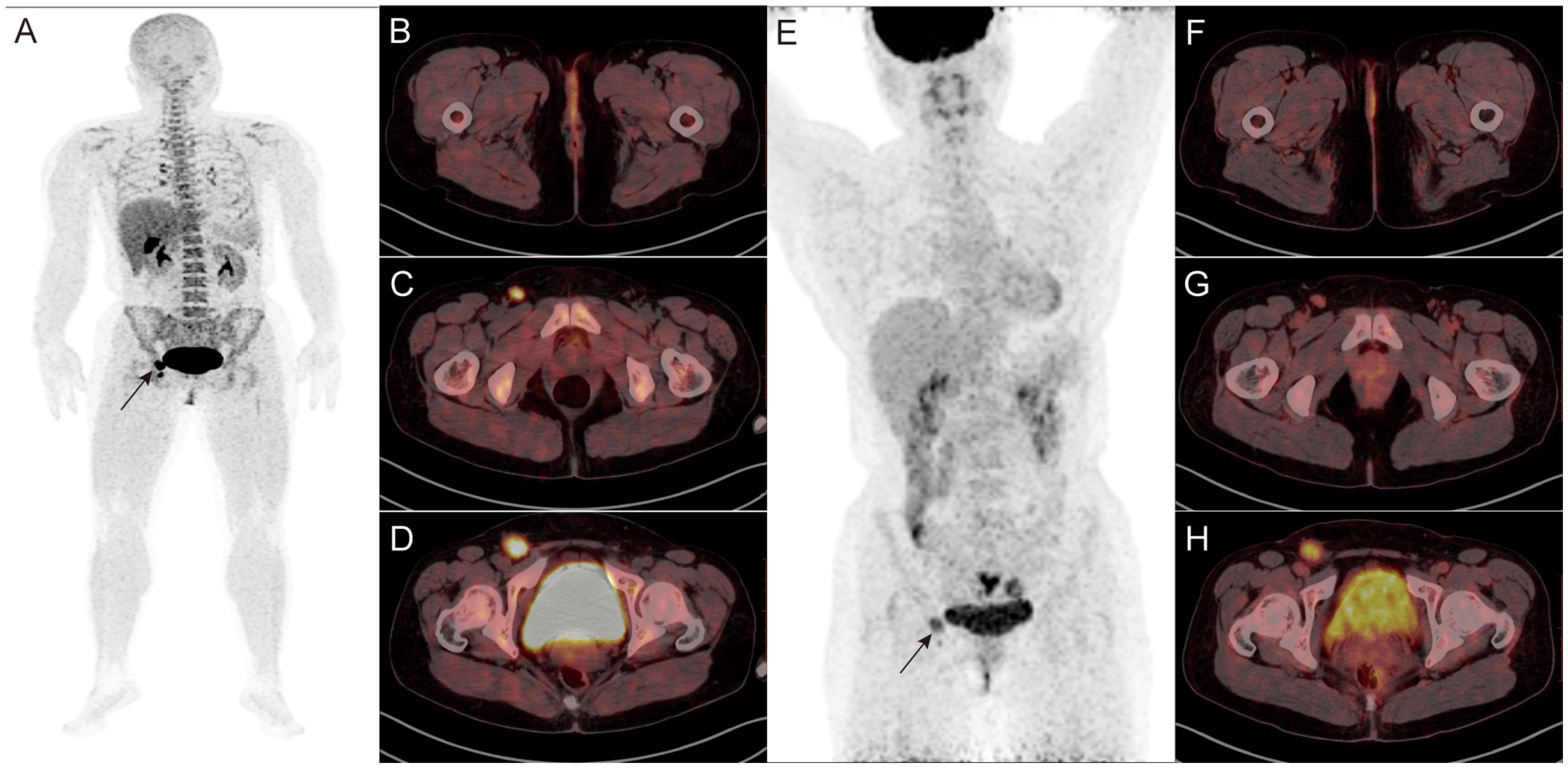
A-H: Findings in a 45-year-old female patient who underwent surgery for external vulvar melanoma 1 year before the start of the study. ^18^F-P3BZA PET/CT MIP (A) showing a radioactive accumulation shadow in the right inguinal region (black arrow). Axial PET/CT (B–D) showing no significant morphological changes or abnormal uptake in the surgical area, with two enlarged lymph nodes with intense radioactive uptake in the right inguinal region (SUVmax: 7.2 and 14.4, respectively). (E–H)
